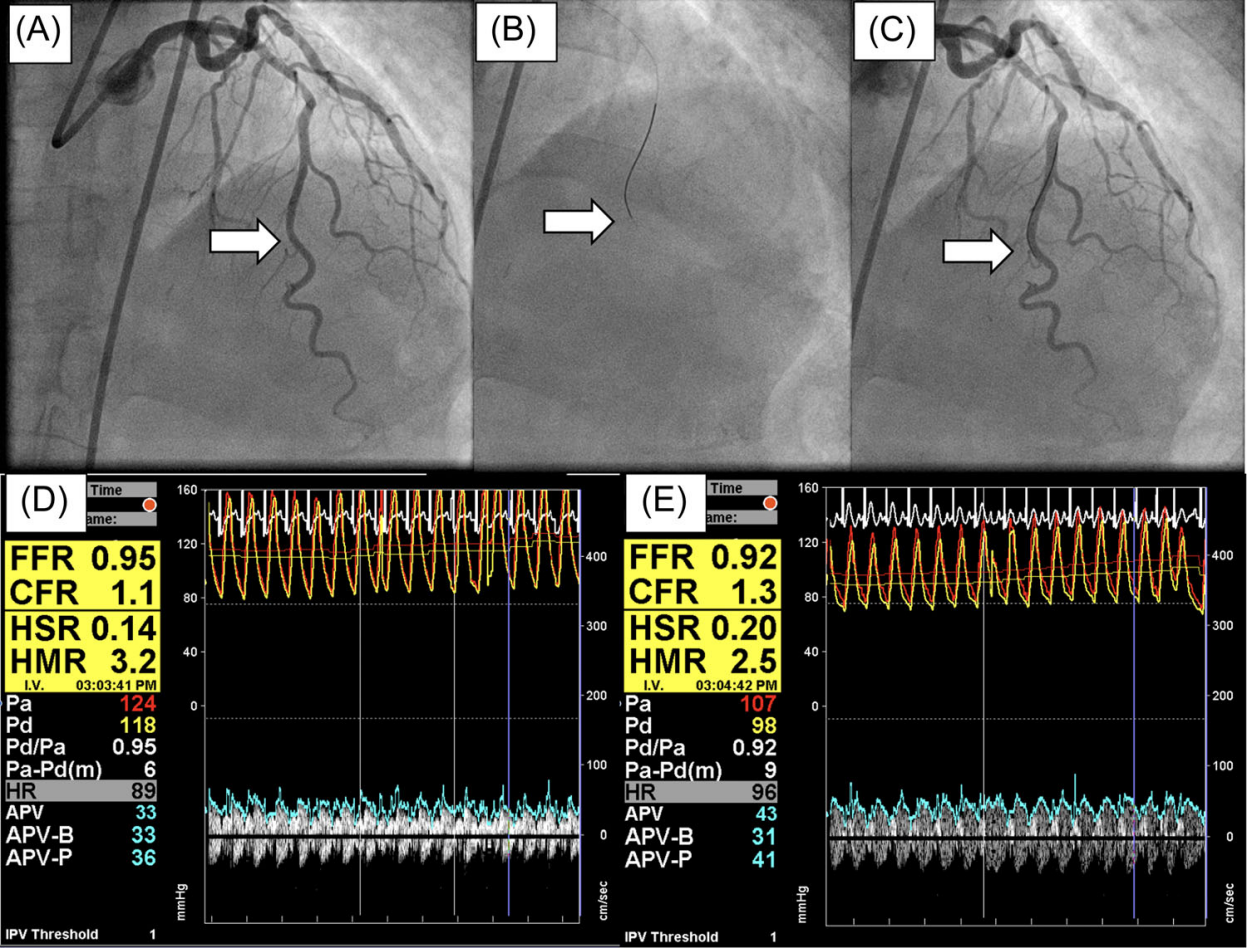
Calculation of coronary physiological values. Hyperemic microvascular resistance index (hMVRI) was calculated as distal coronary pressure (Pd) divided by distal average peak velocity (APV) at maximal hyperemia. Vessel diameter was analyzed by quantitative coronary angiography (QCA) at the site of blood flow measurement by wire (white arrow). QCA was performed with a validated densitometric analysis system (Pie Medical Imaging B.V, Maastricht, the Netherlands). Coronary blood flow (CBF) was calculated as πD^2^/4 (0.5 × APV), where D is vessel diameter. CBF as measured by QCA is described as CBF_QCA_, then hMVRI_QCA_ is calculated as Pd divided by CBF_QCA_ during maximal hyperemia. As CBF_QCA_ will be overestimated, hMVRI_QCA_ will be higher than the original HMR. (A) Coronary angiography. (B) Insertion of the Doppler flow guidewire into the left anterior descending artery. (C) Final position of the Doppler flow guidewire as confirmed by contrast injection. (D) Physiological measurements at rest. (E) Physiological measurements under hyperemic conditions. CFR, coronary flow reserve; HMR, hyperemic microvascular resistance; HSR, hyperemic stenosis resistance.
